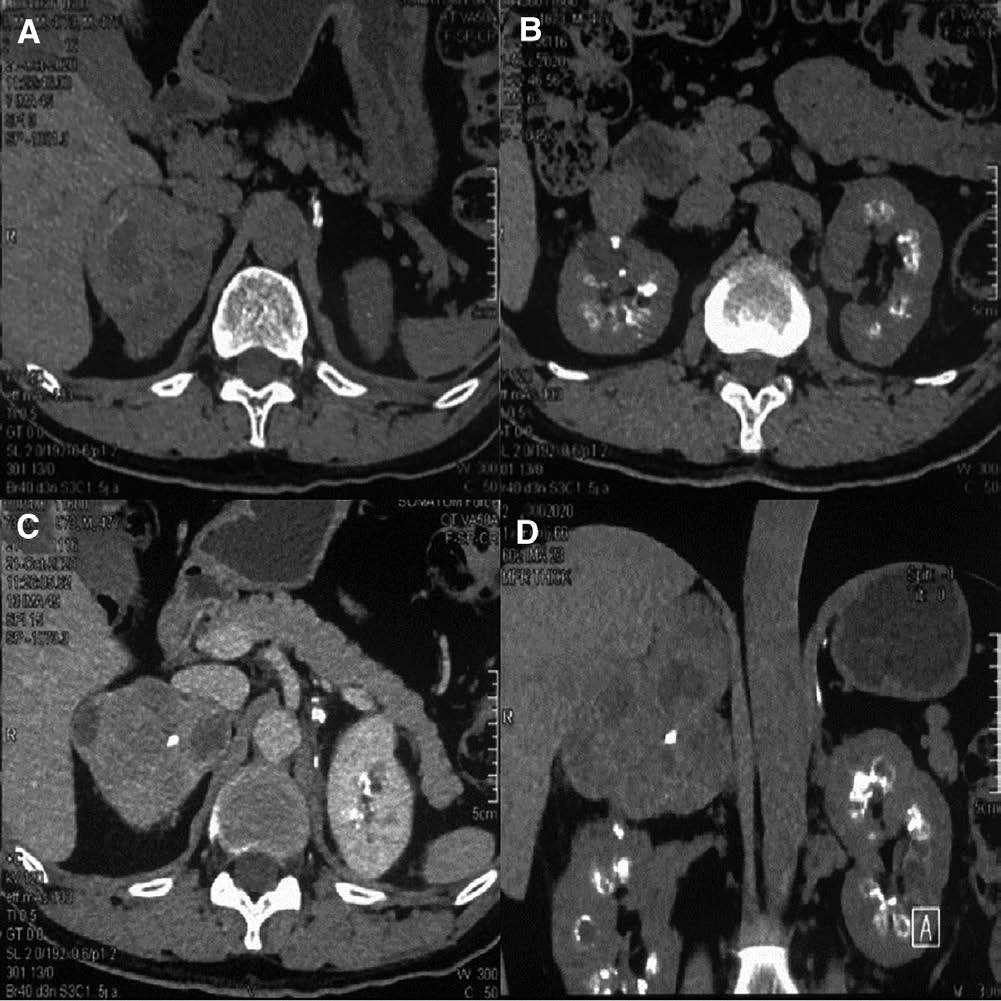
Preoperative reexamination images for the second operation. (A and B) adrenal CT plain scan images, (C) an enhanced CT image of the adrenal gland (post operation on the left adrenal gland, right adrenal pheochromocytoma), (D) a coronal CT scan image (a large mass in the right adrenal region, bilateral medullary spongy kidneys). CT = computed tomography.
